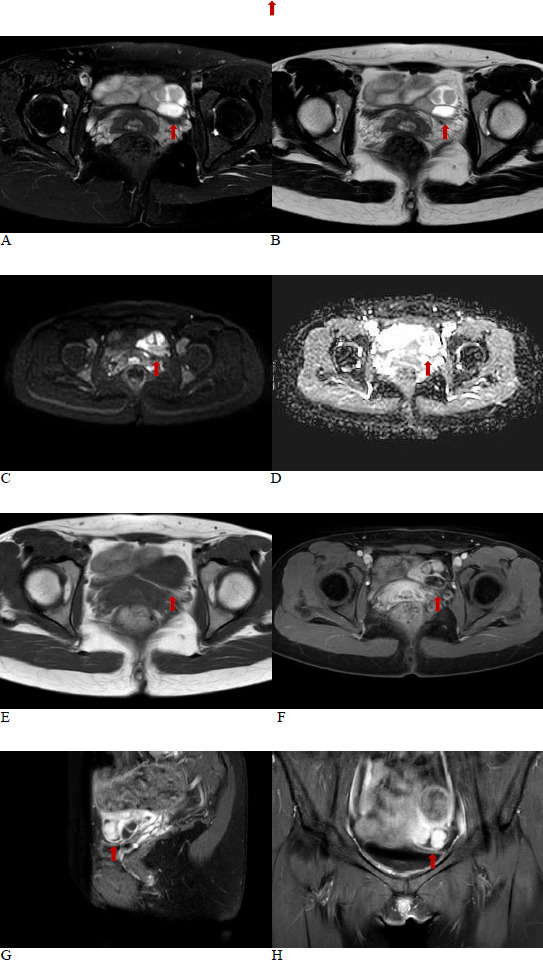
(A,B). Around the left adnexa, a cystic-solid lesion was observed, which showed heterogeneous high signal intensity on T2WI. The fat-suppressed T2WI sequence did not show any reduction, suggesting the absence of fat components. The left ovary was visible, with indistinct margins between it and the lesion. (C,D). The lesion showed high signal intensity in the solid component on diffusion-weighted imaging (DWI), and the ADC signal was reduced, indicating restricted diffusion. (E,H). The lesion showed iso-signal intensity on T1-weighted imaging (T1WI), and after enhancement, the solid component exhibited significant enhancement.
